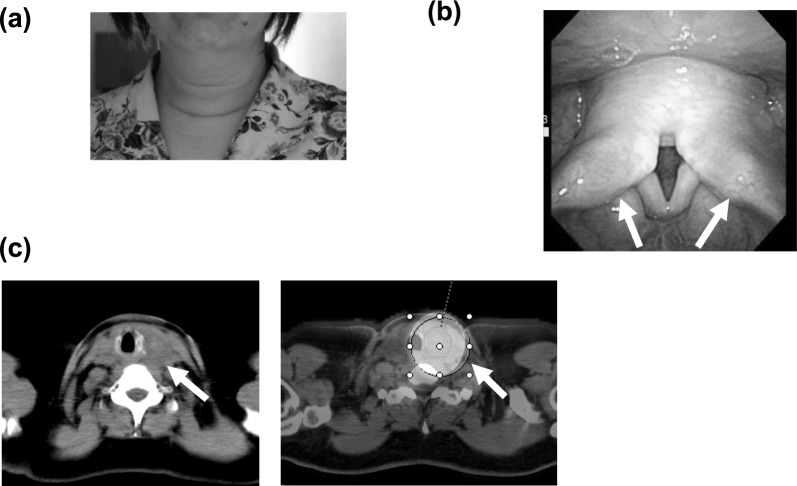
Patient 2, a 54-year-old woman. a Visual inspection 2 days after RAI confirmed swelling in the neck. b Laryngoscopy performed 3 days after RAI revealed edema in the bilateral arytenoid regions and vocal cords. c A CT scan shows mild edematous appearance of the residual thyroid tissue. A SPECT/CT scan demonstrated intense uptake in the residual thyroid tissue
